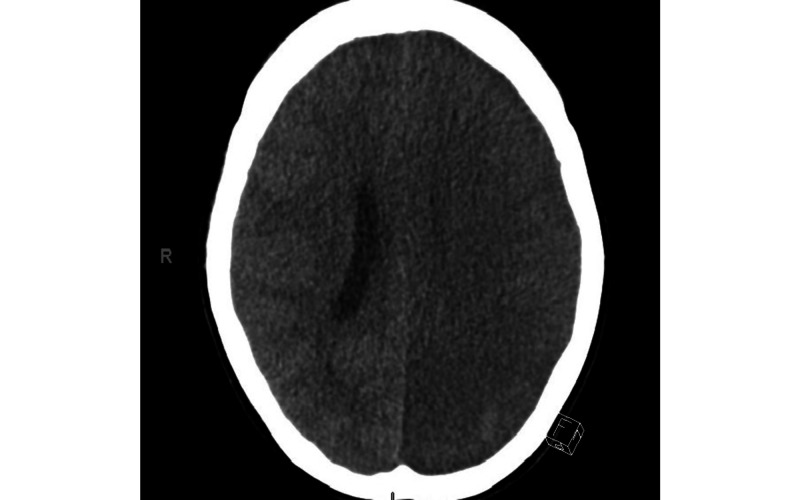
CT scan of the brain showing diffuse cerebral edema with effacement of the basal cisterns and 5-mm rightward midline shift with concerns for impending herniation.
